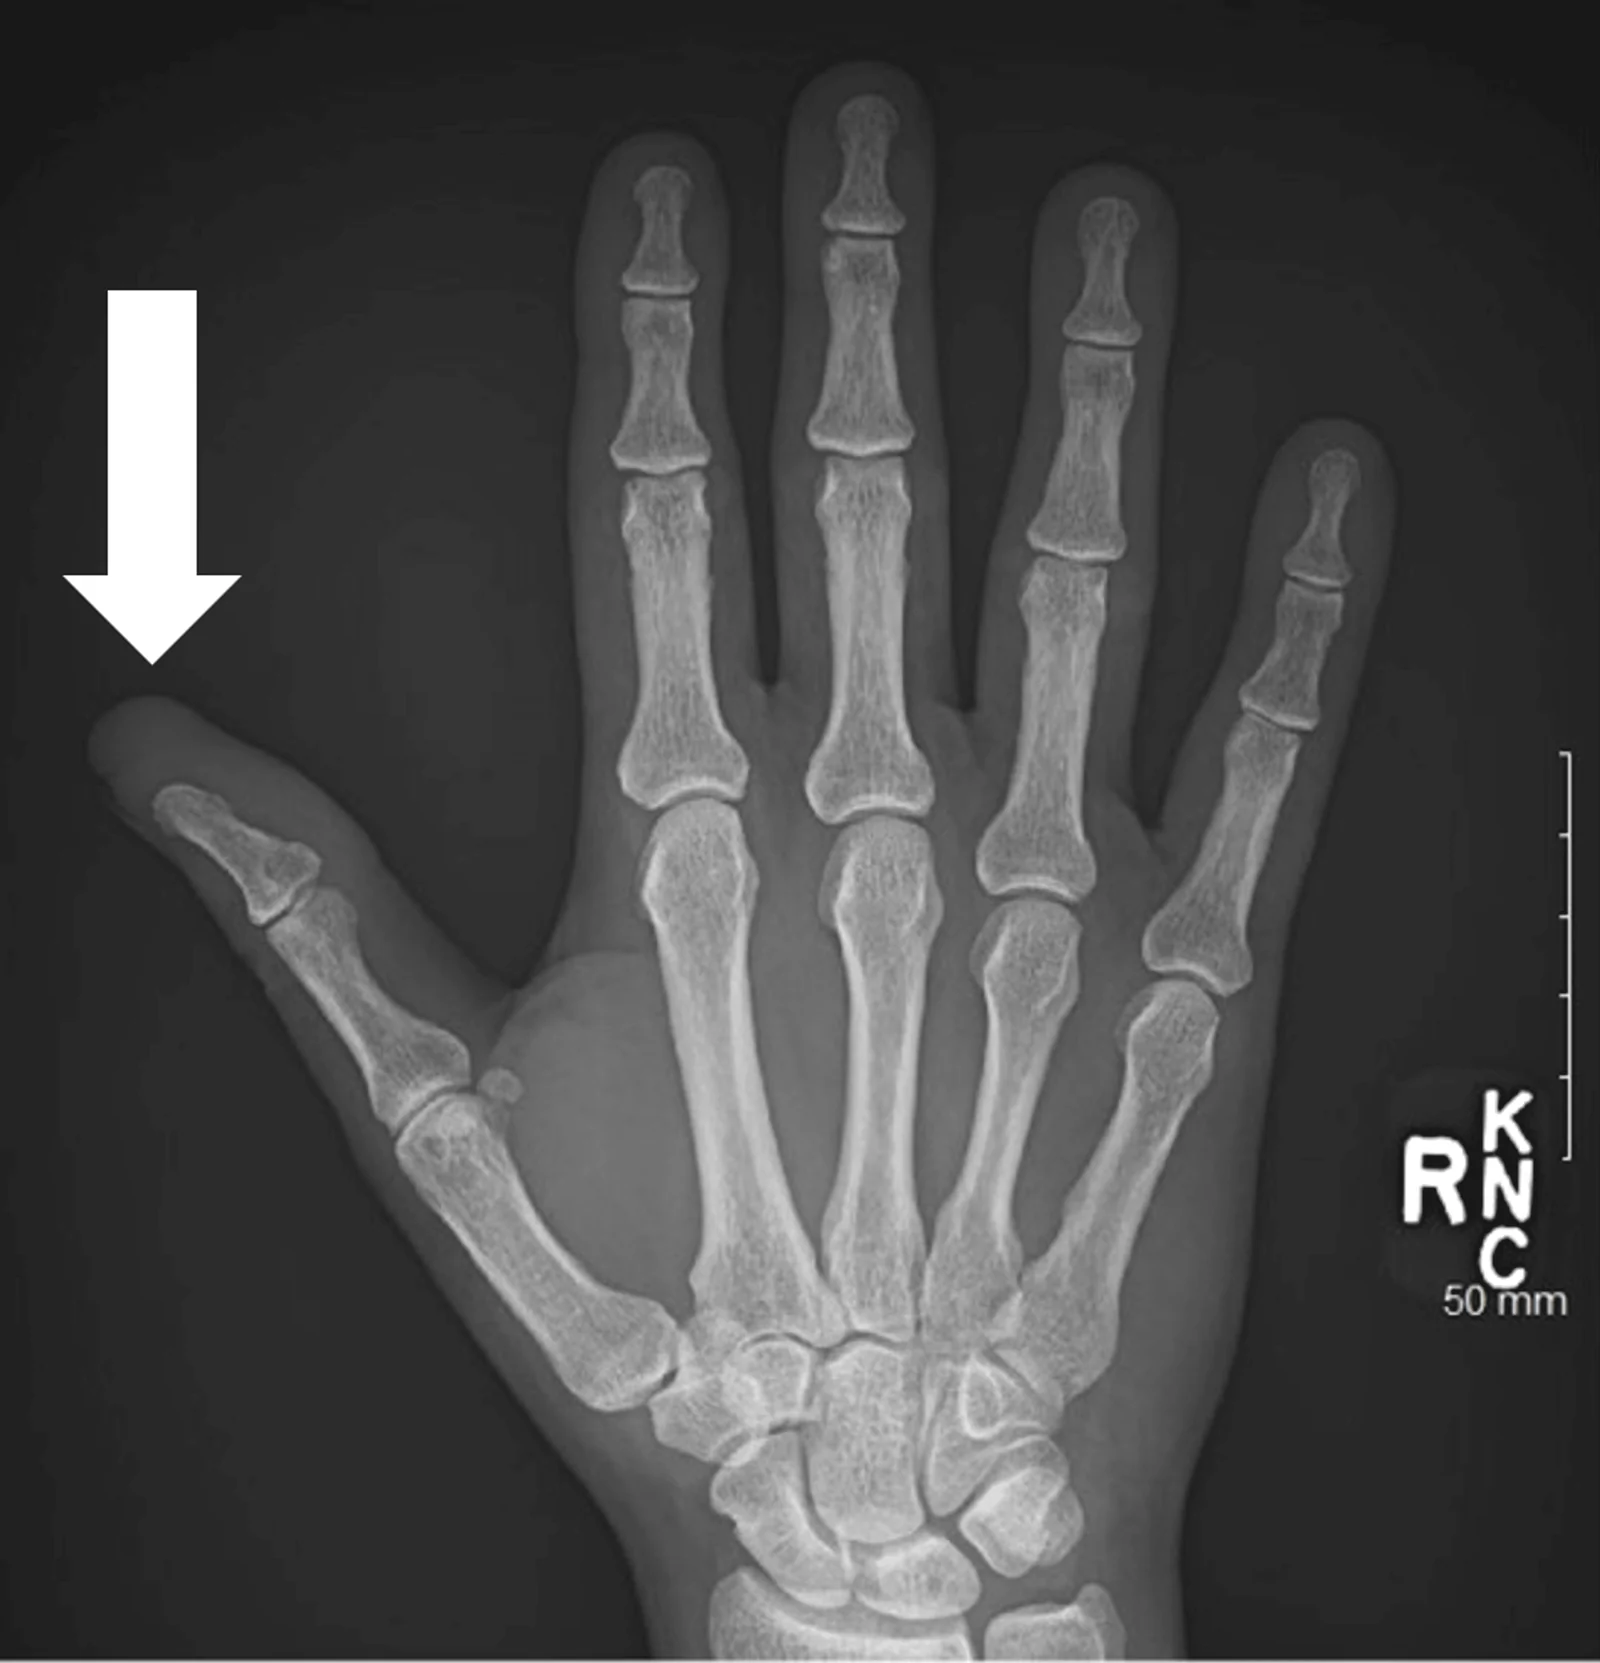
Anteroposterior radiograph of the right hand demonstrating a soft tissue mass at the volar thumb pulp.
